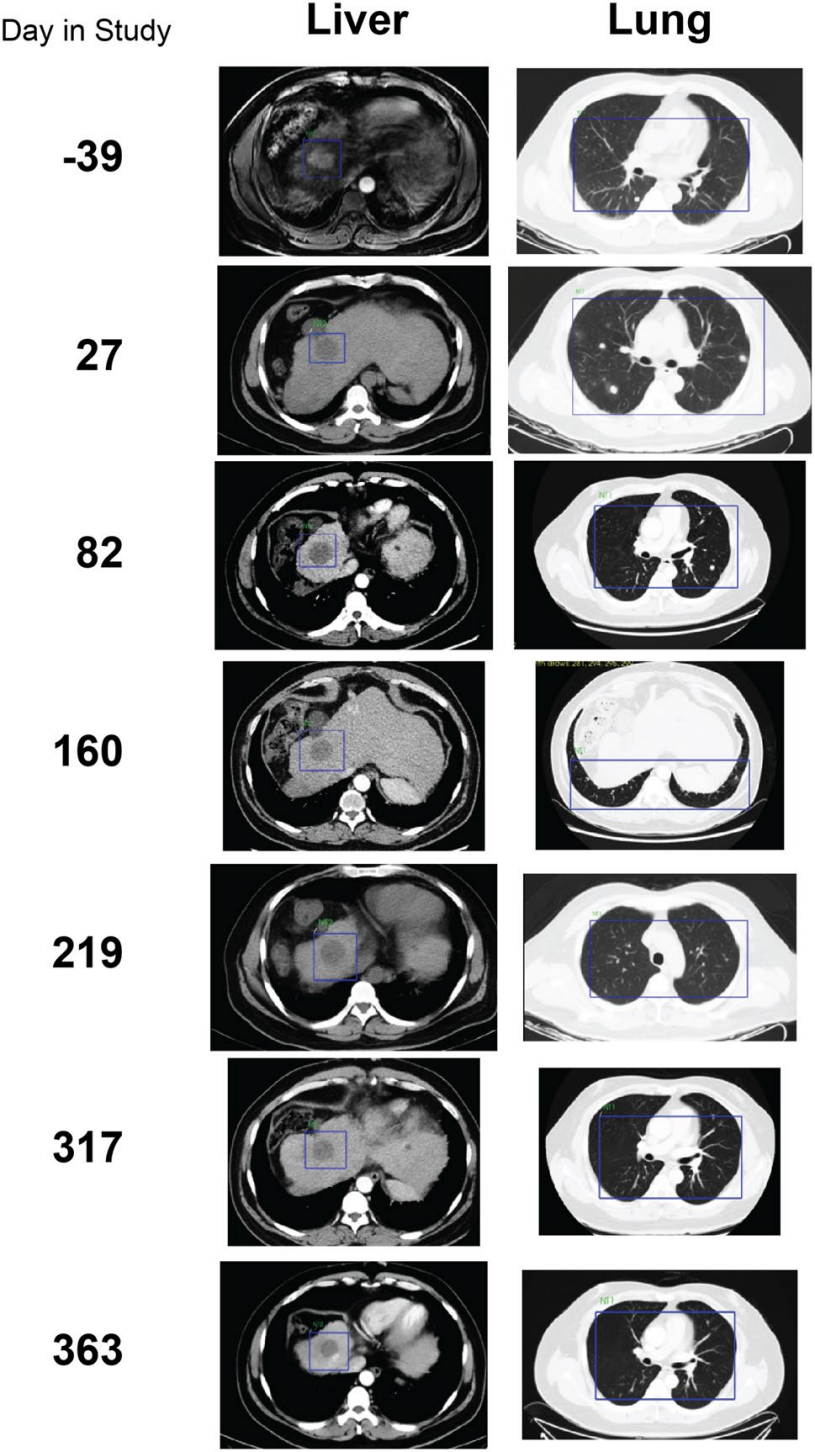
Regression of primary tumor and metastases in Patient 6. A primary tumor is visible in the liver prior to the study start (blue box, day -39) but only the tumor-free cavity remains in subsequent images throughout the course of the study. In the lung, multiple metastases present as white spots throughout the first 82 days of imaging but are undetectable by day 160 and remain so for the remainder of the study. All images are MRI with the exception of day −39 for the liver, which is CT.
